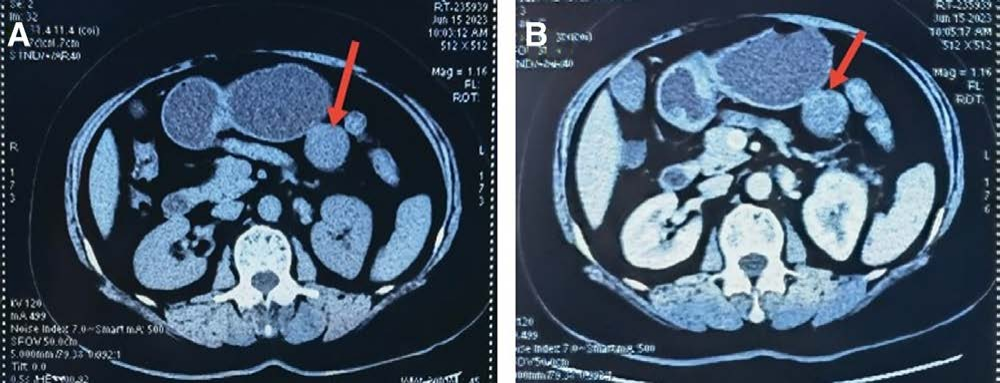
(A) A rounded, slightly hypodense mass was observed in the left abdominal cavity, with a CT value of about 22 HU. The upper edge of the mass was indistinctly demarcated from the greater curvature of the upper part of the gastric body. (B) A shadow of a less homogeneous mass was noted on the left side of the abdominal cavity, predominantly slightly hypodense with a well-defined border. The mass measured approximately 4.0 × 3.8 × 4.5 cm. The enhancement was less than homogeneous, with delayed enhancement visible in local areas. A blood vessel supplying the mass was identified, originating from the inferior mesenteric artery. The lesion was located close to, and was not clearly separated from, the greater curvature of the stomach.
